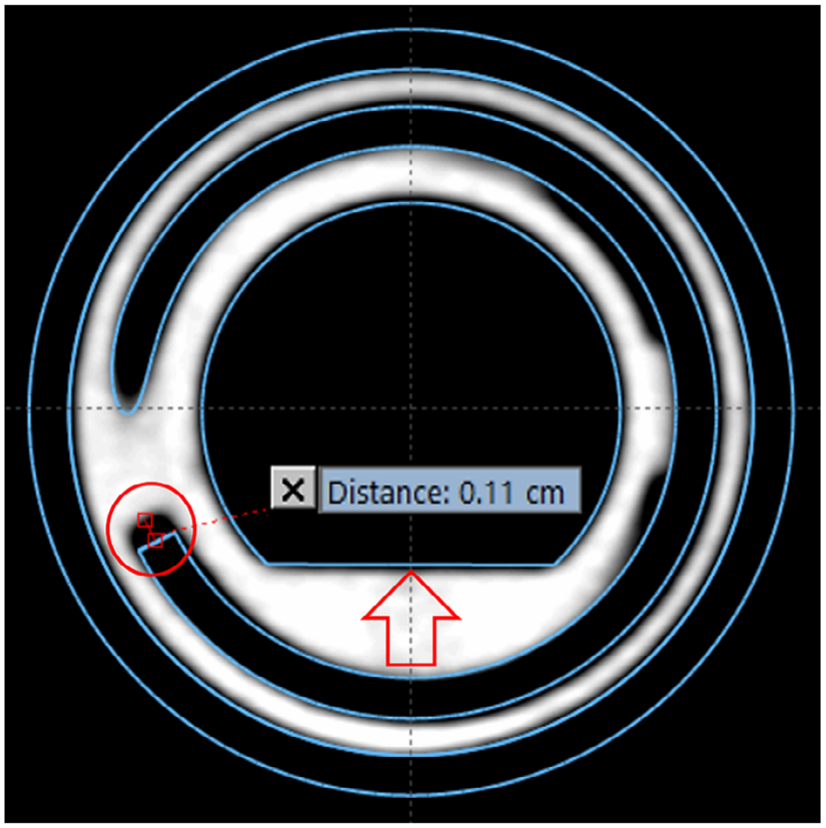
Registration between CT images and solid model was done based on the outer surface of the ring. In particular, angular orientation was determined by matching the straight part of the applicator (marked with a red arrow). A discrepancy of about 1 mm in the position of the lumen tip (marked with a red circle) is clearly visible in the image.
